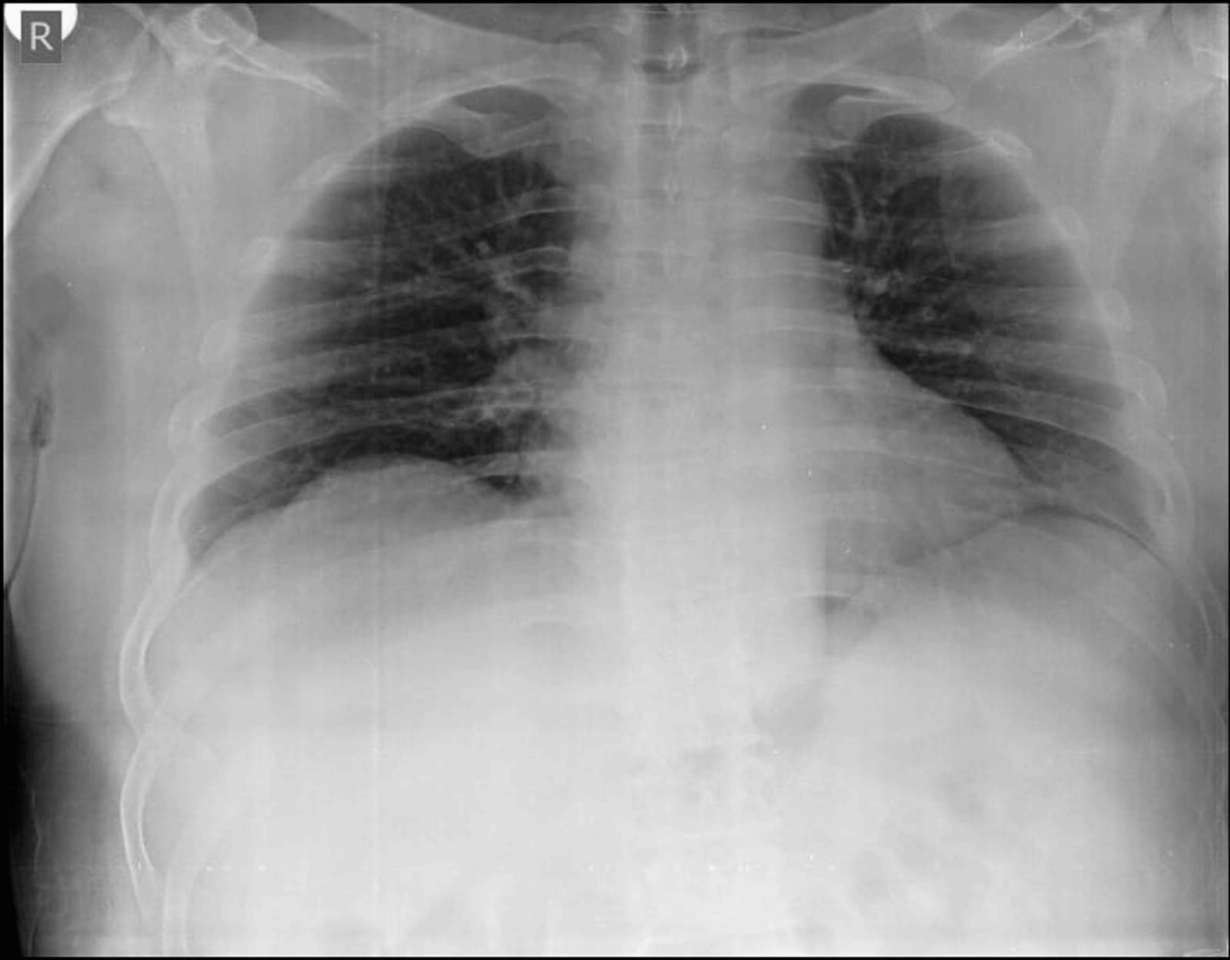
Chest X-ray Posteroanterior projection. In the lower left region, there is a reinforcement of the vascular network and an increase in density suggestive of interstitial infiltrates. The radiologist ruled out pneumonia; findings likely reflect noninfectious pulmonary changes related to Stevens-Johnson syndrome/toxic epidermal necrolysis overlap
